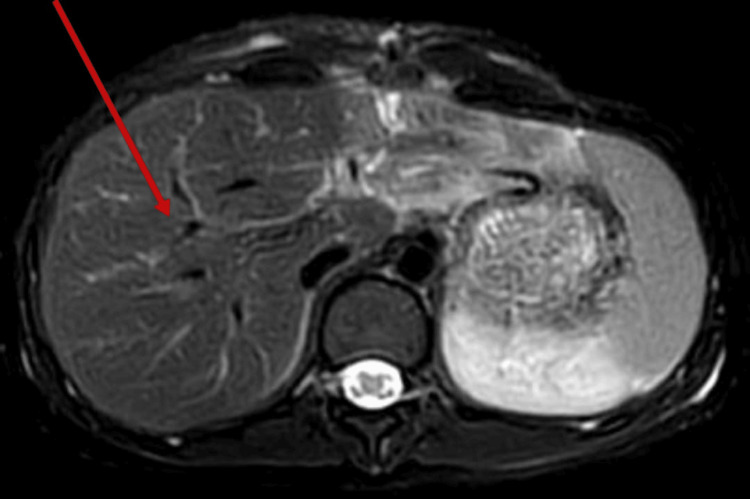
Magnetic resonance image showing a normal right liver lobe
